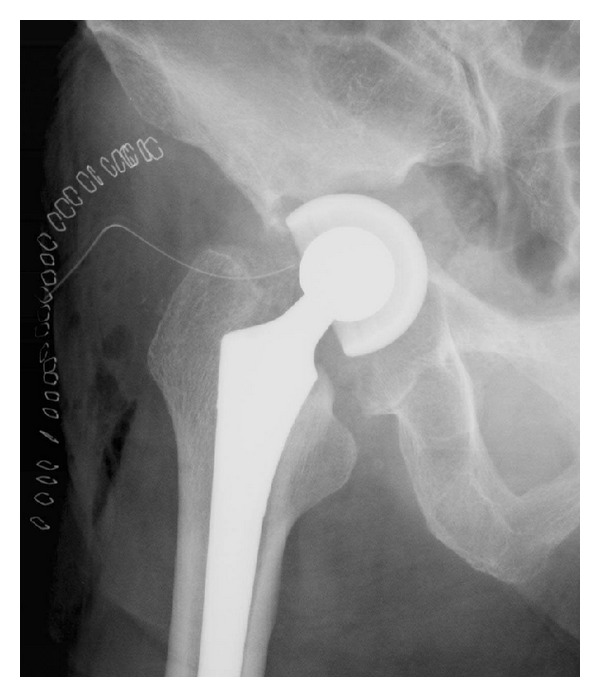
Anteroposterior radiograph showing severe acetabular bone loss.
